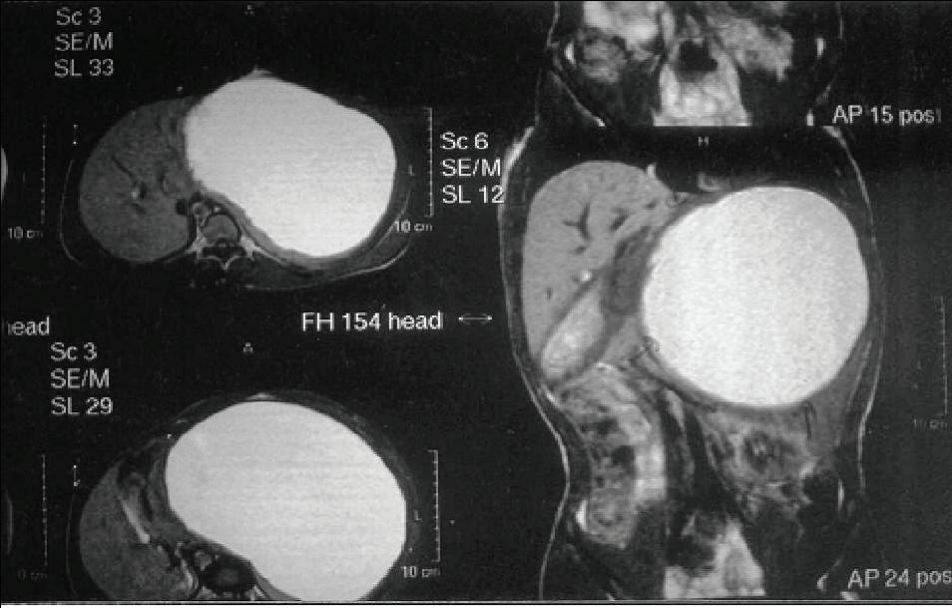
MRI demonstrating a large well-defined, unilocular, cystic, encapsulated lesion of spleen.
